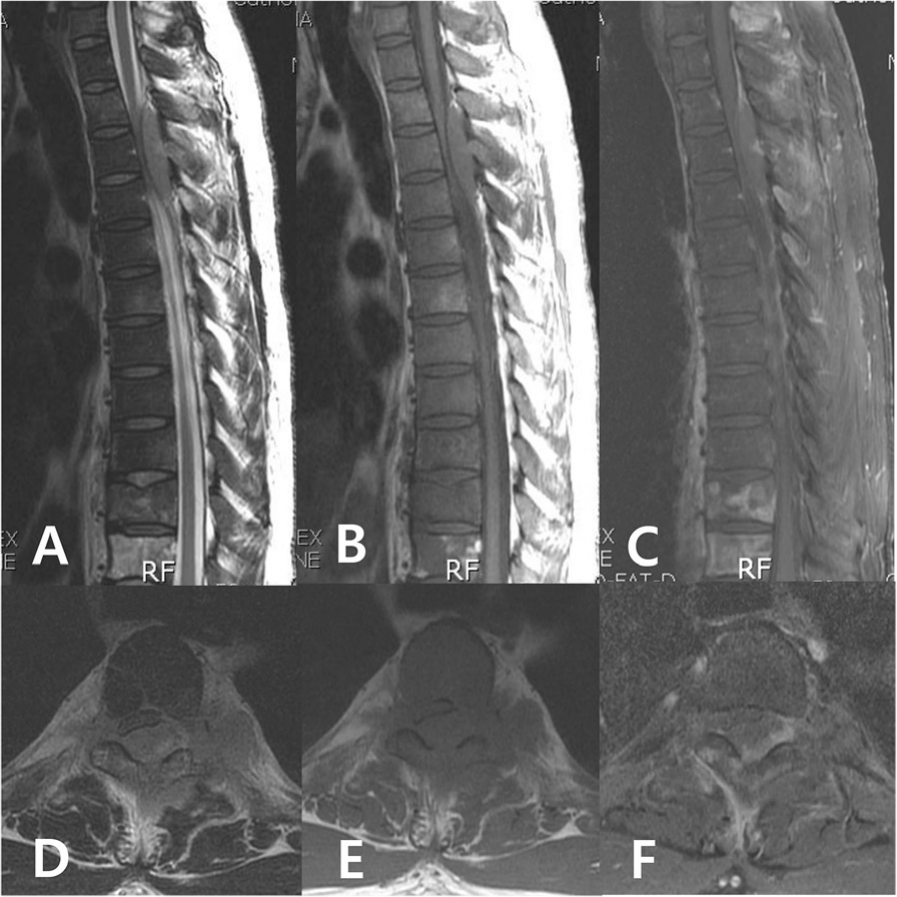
Magnetic resonance image shows elongated epidural mass at the left posterolateral aspect of the spinal cord at the T2 to T4 levels, resulting in severe cord compression. A, T2-weighted image sagittal; B, T1-weighted image sagittal; C, T1-weighted image enhanced sagittal; D, T2-weighted image axial; E, T1-weighted image axial; F, T1-wighted image enhanced axial.
